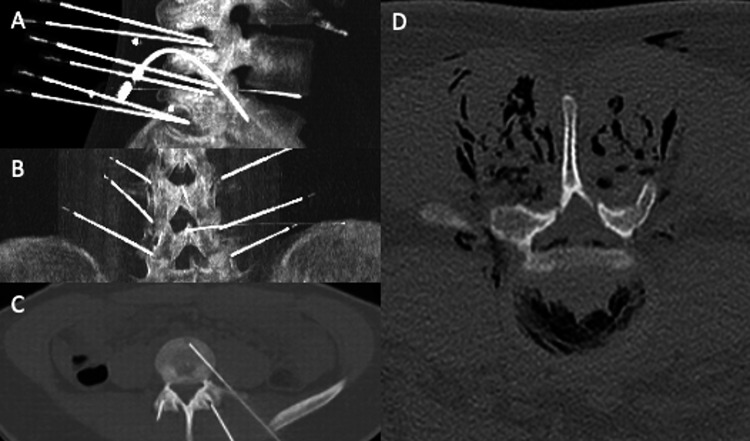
Conventional percutaneous CT-guided injection. The same procedure was done under CT guidance with the needles inserted into the facet joints and into the herniated disc (A–C). At the end of the procedure, a CT scan was performed to confirm the adequate O_2_/O_3_ intradiscal and paravertebral diffusion (D)
